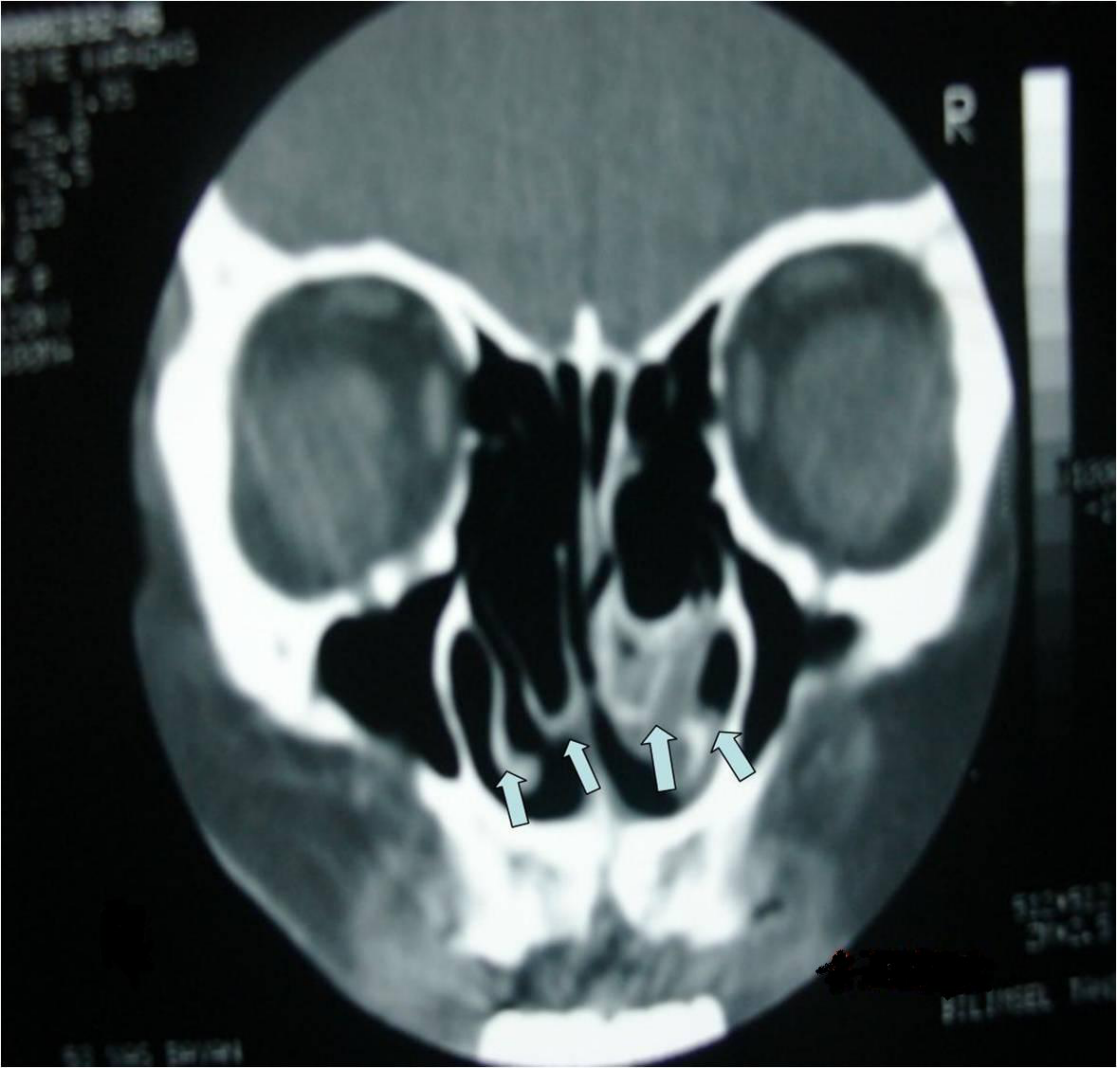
Coronal paranasal computed tomographic scan from a different angle showing a concha bullosa inside the bilateral middle concha.
